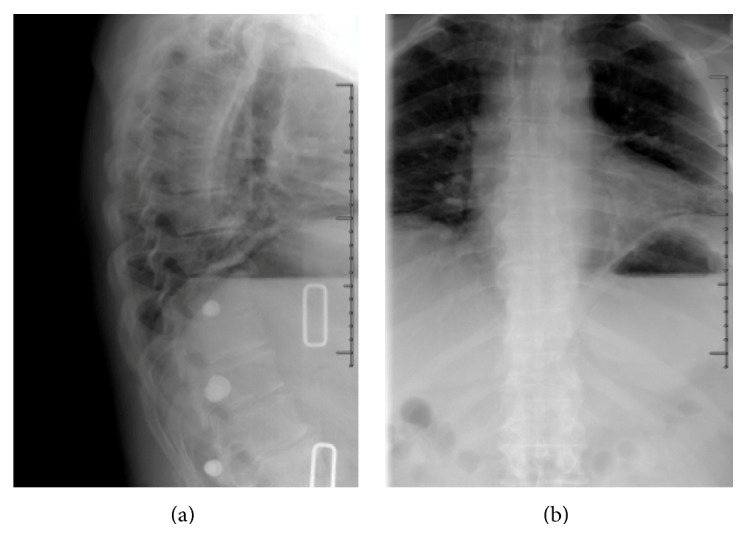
Sagittal (a) and anteroposterior (b) plain film in thoracolumbar sacral orthosis brace at 10 weeks after the initial injury, demonstrating good osseous union and well-maintained sagittal alignment.
